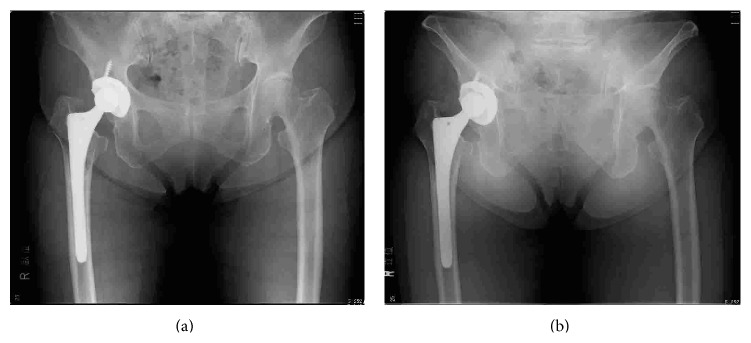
Radiographs of a 72-year-old woman treated with right hip THA. An HXLPE liner was implanted, and the annual polyethylene wear rate was 0.008 mm/year. (a) AP pelvic radiograph in the supine position 5 years after THA; cup inclination was 46°, cup anteversion was 16°, and PTA was 31°. (b) AP pelvic radiograph in the standing position shows that the pelvis is tilted posteriorly. The PTA was 59°, leading to an increase in cup inclination and anteversion; moreover, cup inclination was 52° and cup anteversion was 38°.
